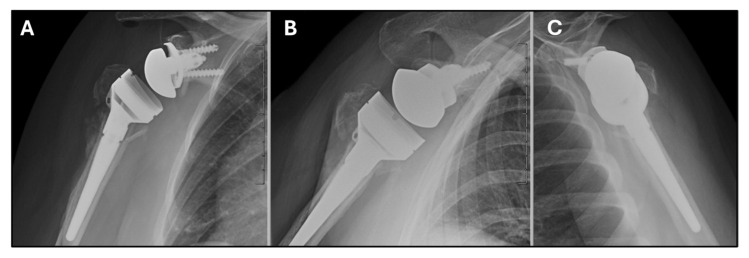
Radiographs of the right shoulder at 6 weeks post-op showing reverse total shoulder arthroplasty with maintained tuberosity reduction and alignment. (A) Grashey view. (B) Velpeau view. (C) Scapular Y view.
